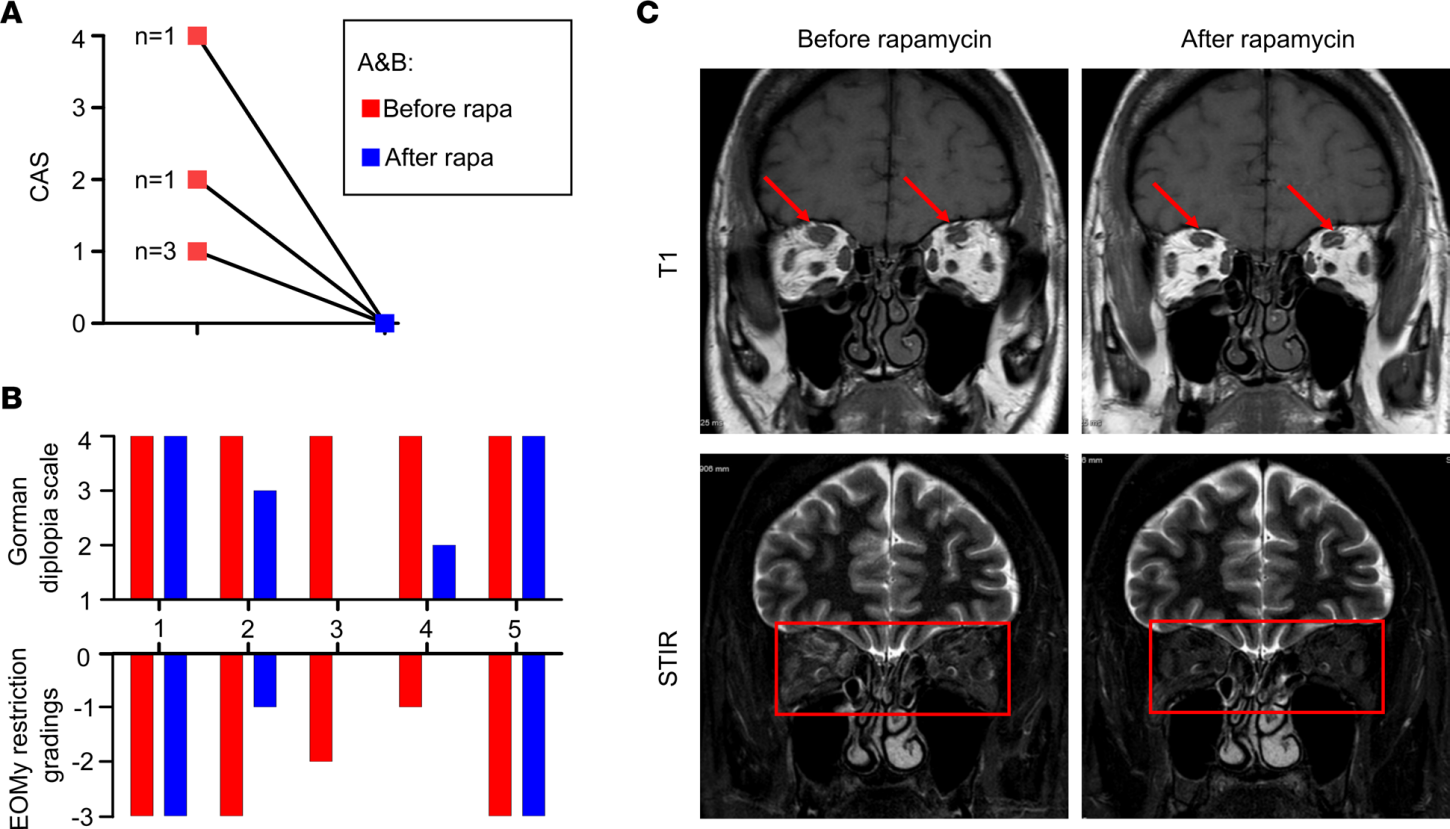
Rapamycin improves orbitopathy and diplopia in patients with refractory GO. (A) CAS before and after rapamycin treatment (*n* = 5). (B) EOMy restriction gradings and Gorman diplopia scale of individual patients with GO. (C) Representative MRI images on T1 weight and short tau inversion recovery (STIR) sequence of good responders before and after rapamycin treatment.
